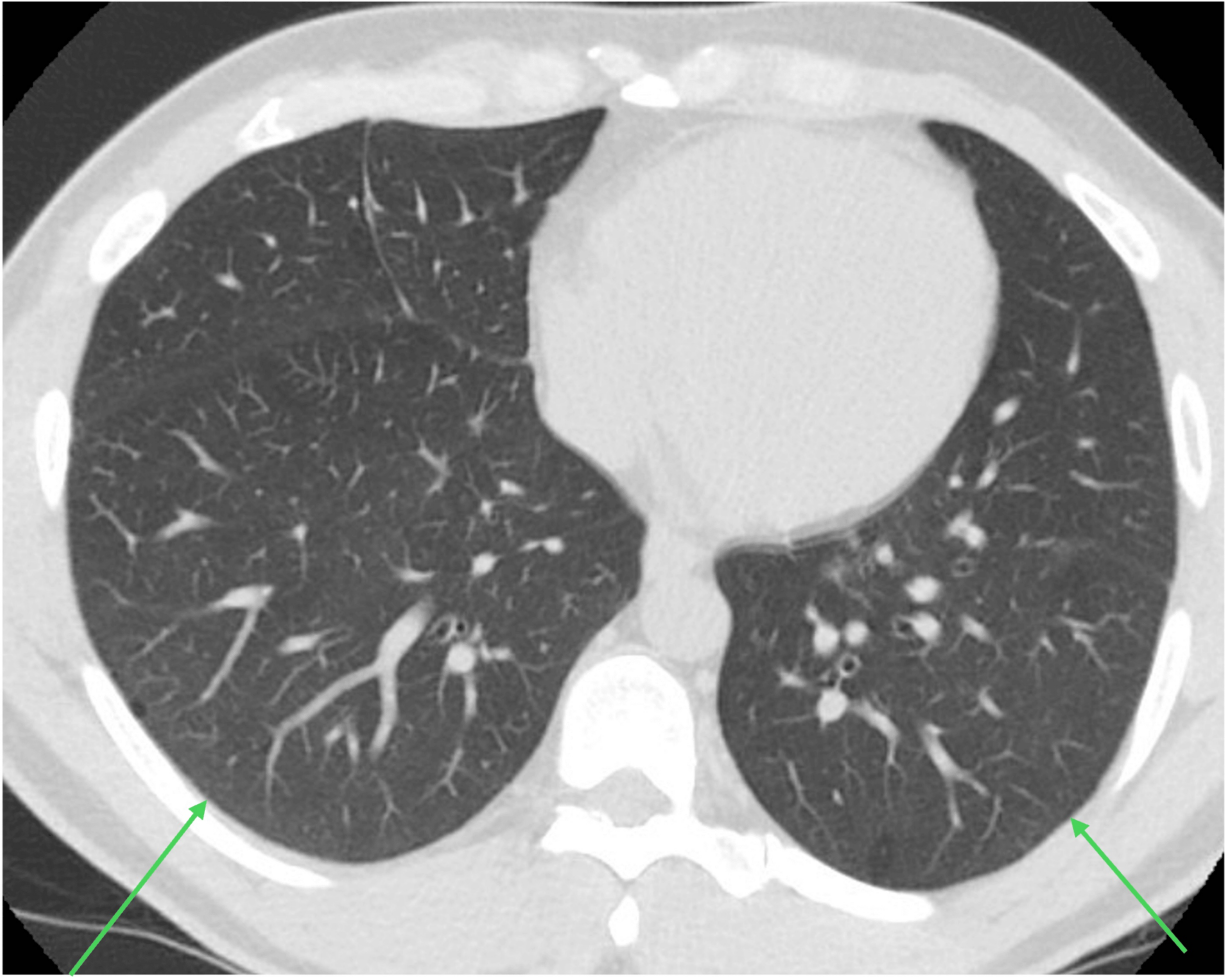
CT at eight months showed an interval resolution of previously noted GGO in both lungs GGO, ground-glass opacities.
